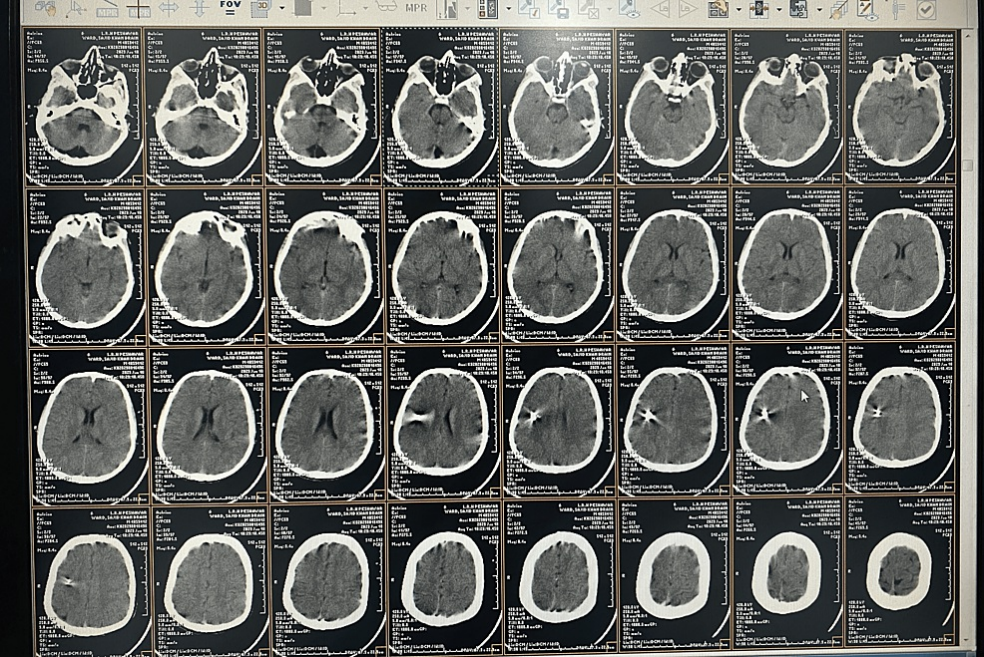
Computed tomography image The foreign body with a ring shape and metallic glare can be seen on the CT scan in the frontal lobe region near the cortex.
